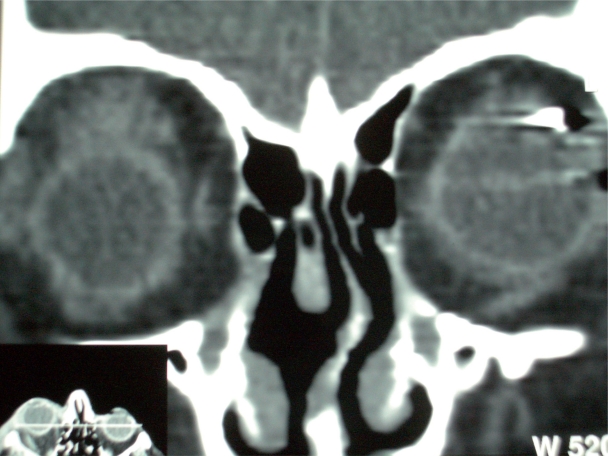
CT Scan Orbits: mass in right orbit.
